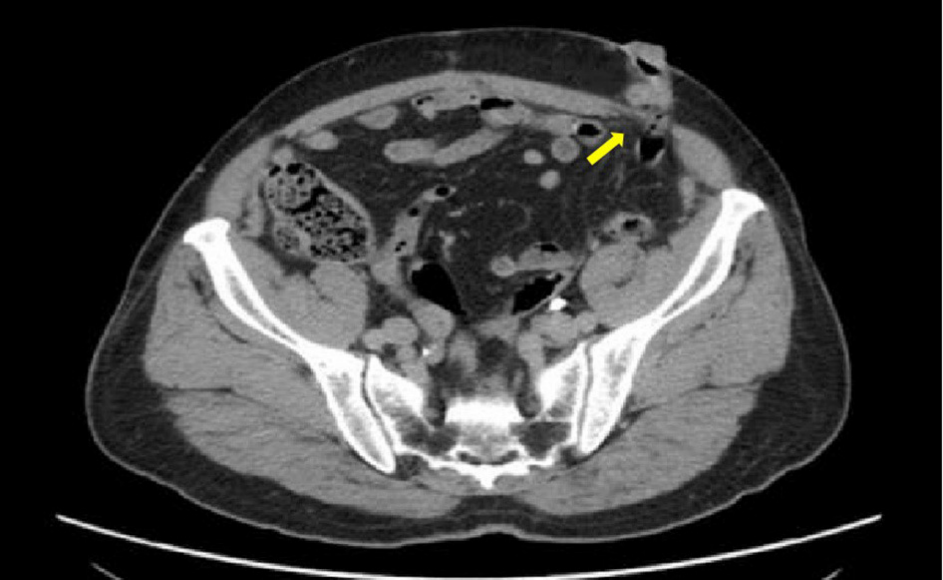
Post-operative CT image showing no recurrence of hernia (yellow arrow).
